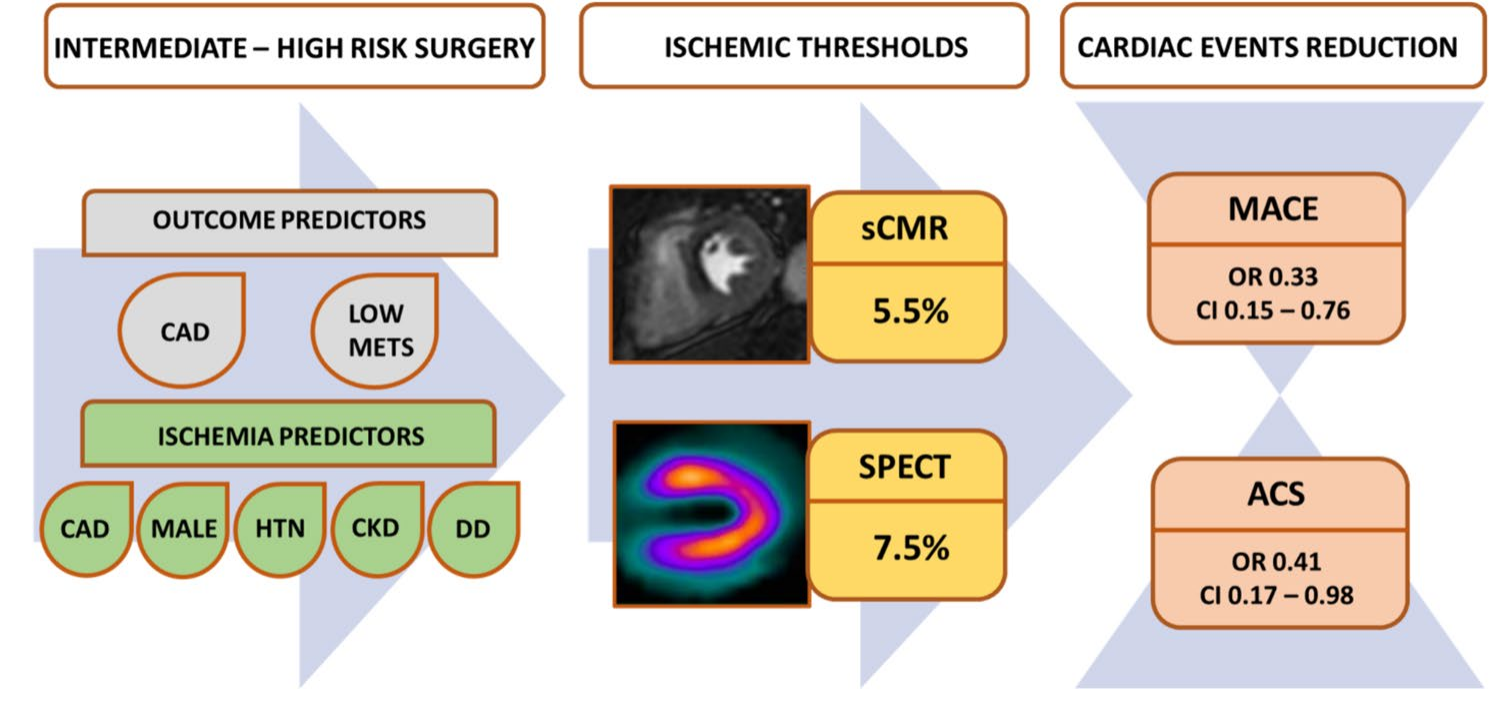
Proposed algorithm to select patients who may benefit from a pre-operative stress test strategy. Both outcomes predictors and ischemic predictors must be considered during the preoperative evaluation before NCS. The suggested ischemic thresholds are 5.5% for sCMR and 7.5% for SPECT. The imaging stress test strategy is associated with a reduction in both MACE and ACS in the 30-day postoperative period. *ACS* acute coronary syndrome, *CAD* coronary artery disease, *CI* confidence intervals, *CKD* chronic kidney disease, *DD* diastolic dysfunction grade II and III, *HTN* hypertension, *MACE* major adverse cardiac events, *METS* metabolic equivalents, *NCS* non-cardiac surgery, *OR* odds ratio, *sCMR* stress cardiac magnetic resonance, *SPECT* single-photon emission computed tomography
